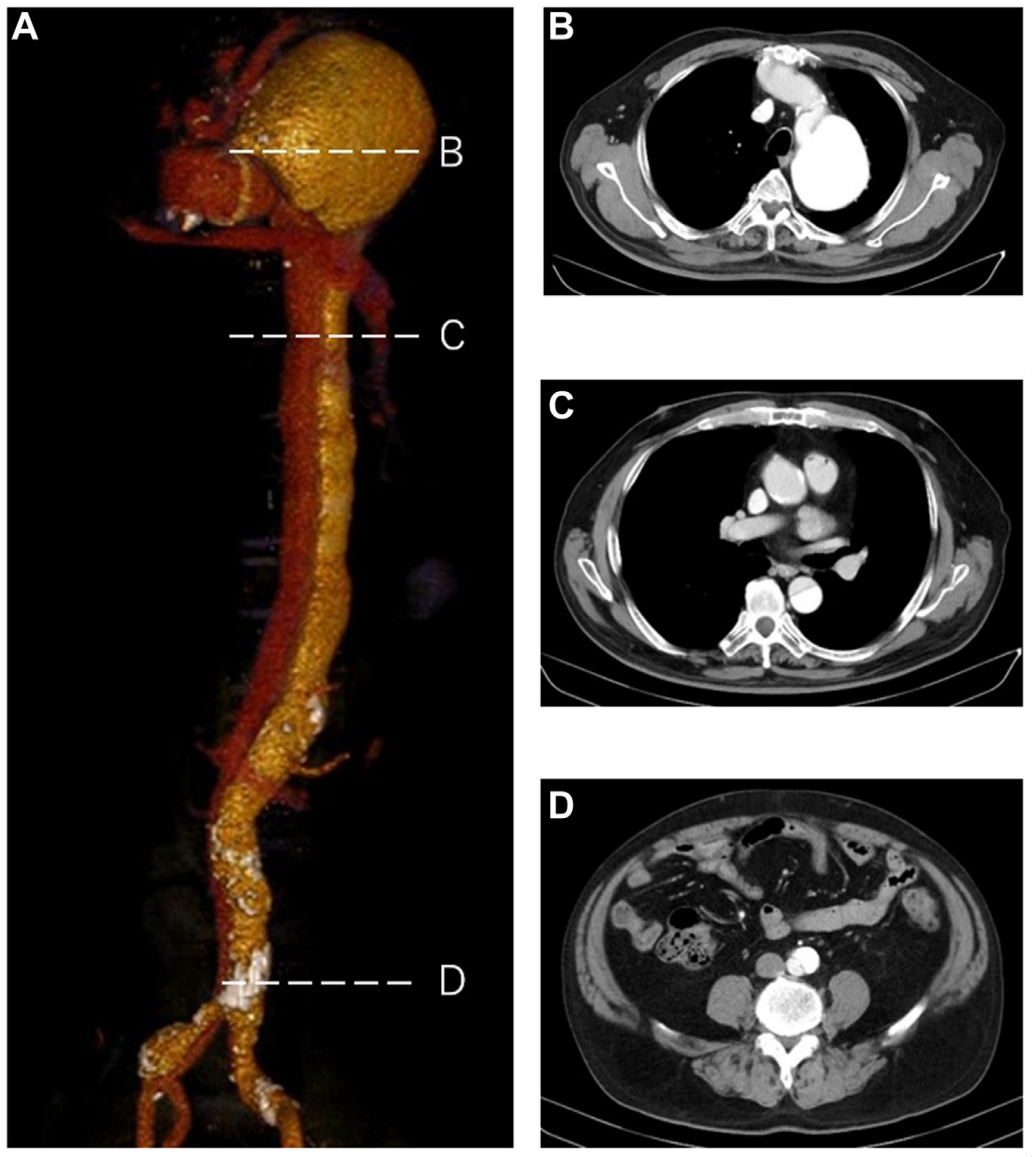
Preoperative contrast computed tomography (CT) scan. (A) A three-dimensional (3D) reconstruction of a preoperative computed tomographic image showing an aortic aneurysm of the distal aortic arch associated with a patent false lumen owing to the intimal tear adjunctive to the elephant trunk. A horizontal view matching the dotted line and the letter is shown on the right. (B) A diameter of 7.4 cm maximum dilatation of the false lumen in the distal aortic arch. (C) Double-barreled thoracic descending aorta. (D) Reentry in the terminal aorta.
